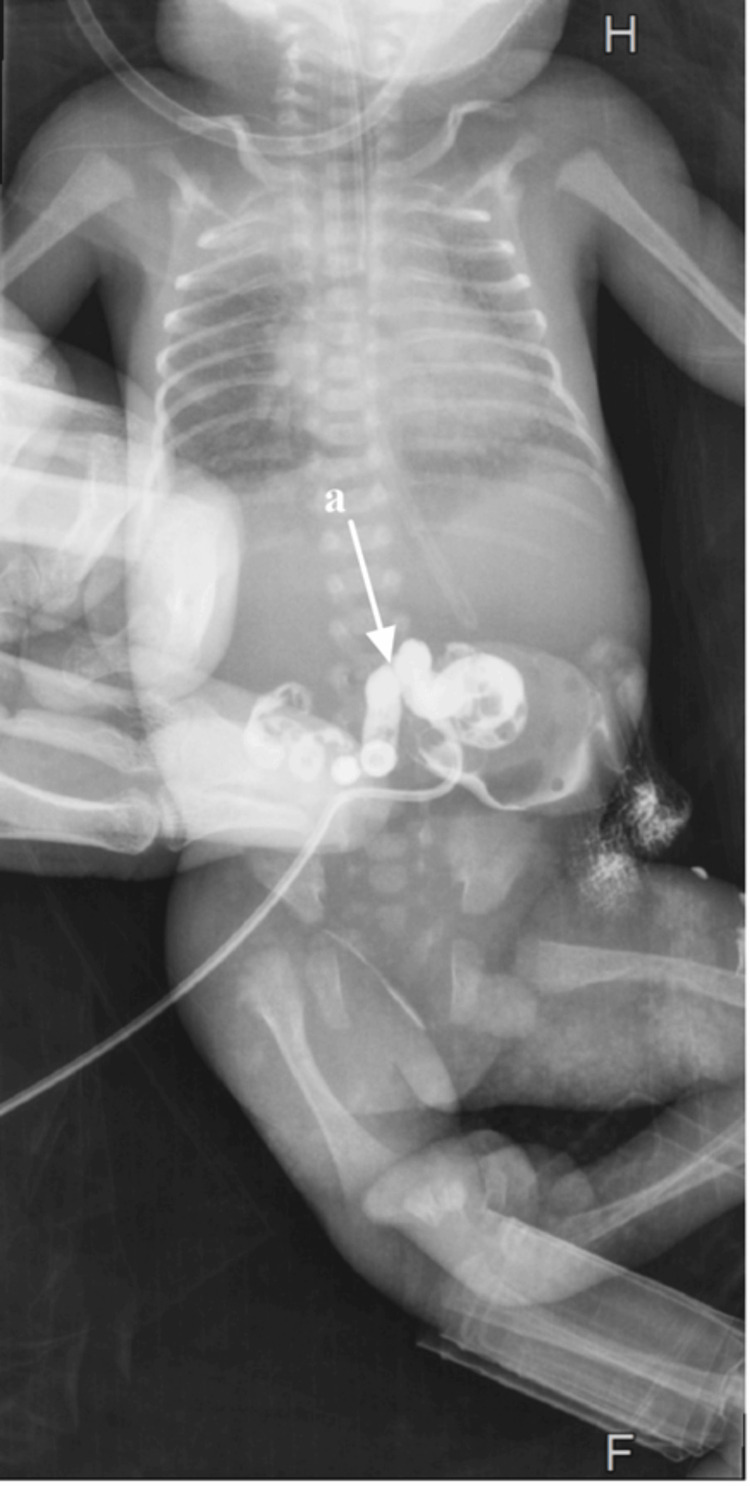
Radiographs with contrast show the continuity of the omphalomesenteric defect with the gastrointestinal tract (a).
